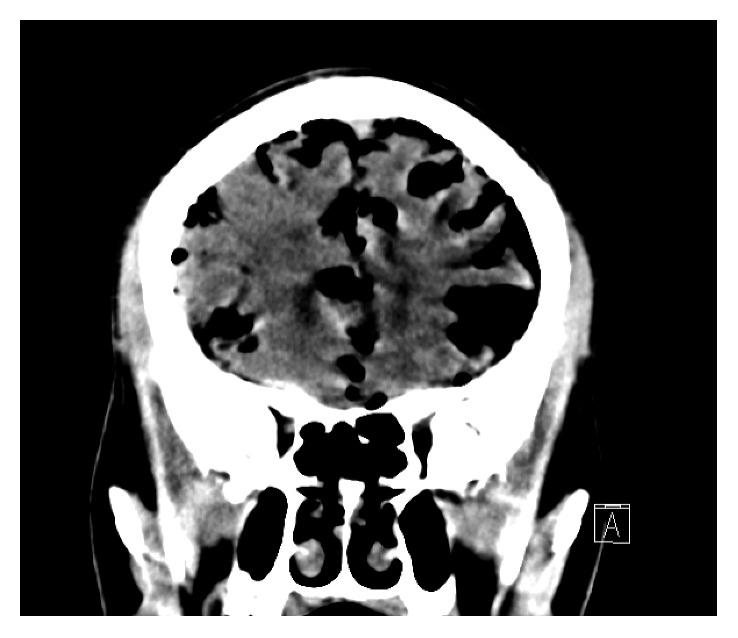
CT head with extensive pneumocephalus within the sulci and cisterns.
